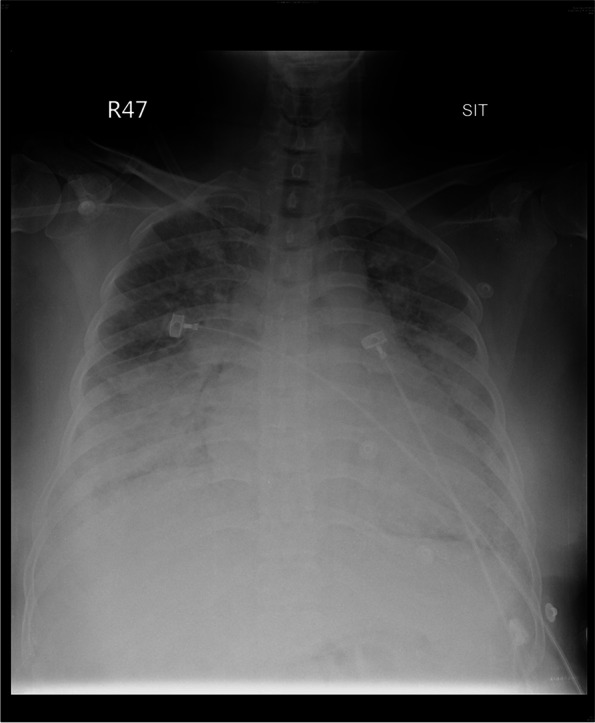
Chest radiography revealed cardiomegaly, bilateral interstitial infiltrates, and patchy opacities (multifocal consolidations)
